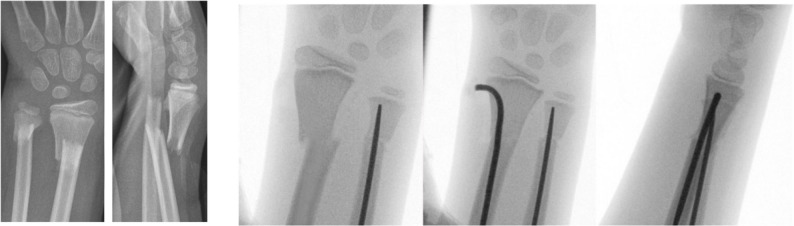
Pre- and intraoperative radiographs of a distal forearm fracture in a 9-year-old child treated with the modified retrograde ESIN technique in the radius and antegrade ESIN fixation of the ulna
